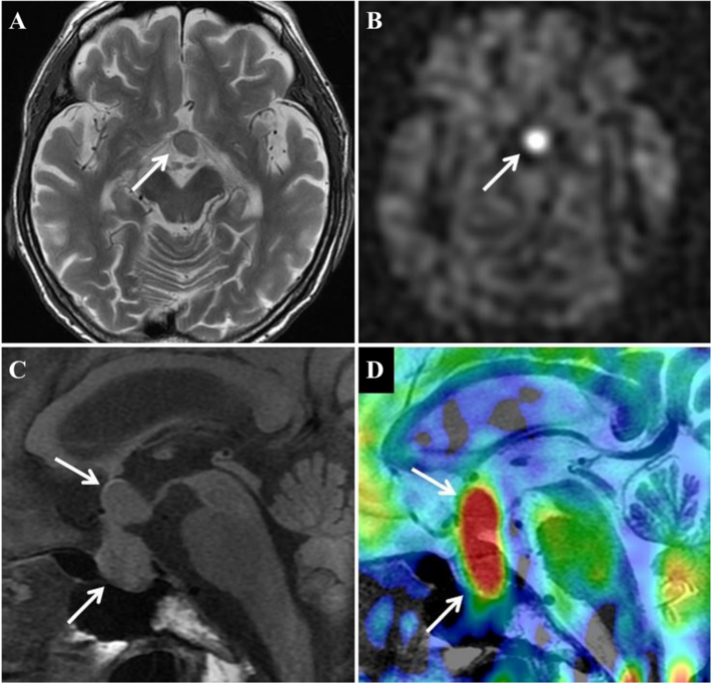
Magnetic resonance images of the patient on admission. (a) Axial T2-weighted image showing a tumor located in supra-sellar region (arrow). The tumor is imaged as same intensity as gray matter. (b) Arterial spin labeling (ASL) perfusion weighted image corresponding to the section of (a). The tumor is revealed as bright hyper-intensity mass (arrow), suggesting elevated blood flow. (c) Sagittal T1-weighted pre-contrast image. The tumor is located in pituitary fossa and had spread across the suprasellar region (arrows). (d) ASL fusion image onto a T2-weighted image. The fusion image demonstrates high blood flow signal exactly corresponds to the tumor mass (arrows).
